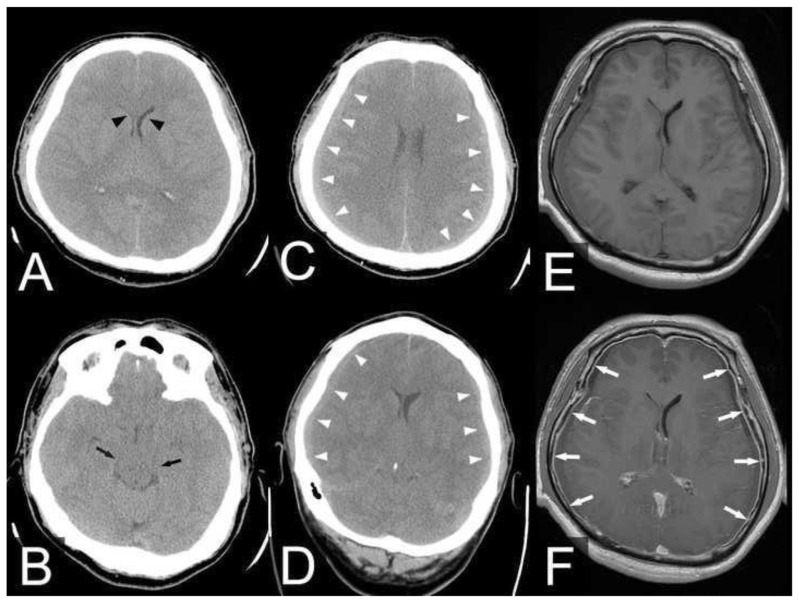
(A, B) Brain CT on Day 1 of hospitalization showed a slit ventricle (black arrowhead) and saggy brain with basal cistern obliteration (black arrow). (C, D) Brain CT on Day 3 of hospitalization showed bilateral subdural fluid collection (white arrowhead) with mass effect. (E) Non-contrast brain MRI T1-weighted image showed bilateral subdural fluid collection. (F) Contrast brain MRI T1-weighted image showed pachymeningeal enhancement (white arrow).
